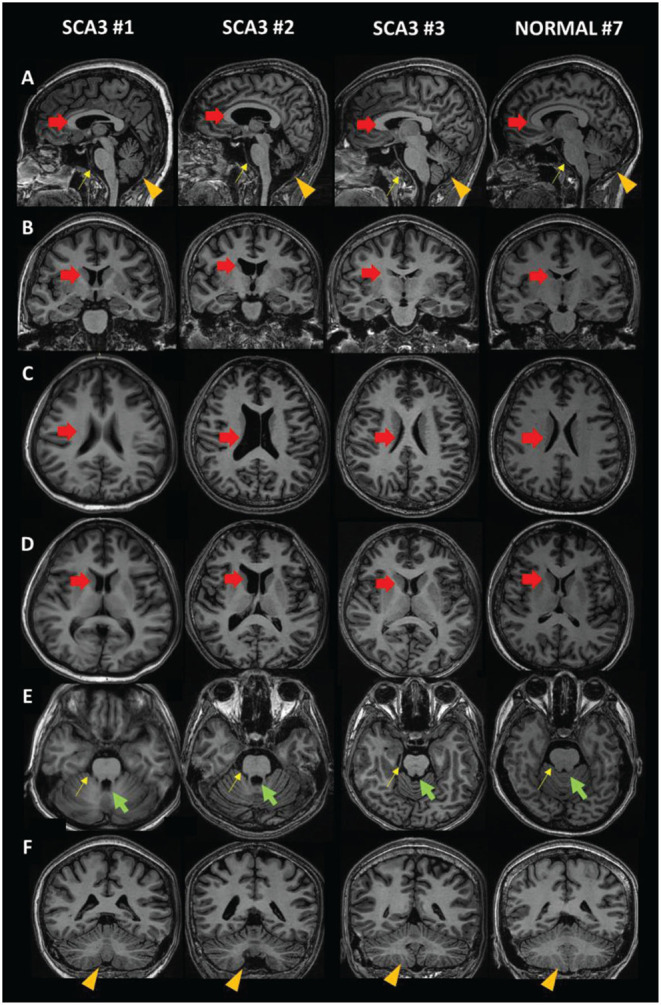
MRI images of SCA3 patients compared to a normal subject. (A) Sagittal view. (B,F) Coronal view. (C–E) Axial view. Red arrow: lateral ventricle (dilated in SCA3); green arrow: fourth ventricle (dilated in SCA3); yellow arrow: brainstem (size reduced in SCA3); orange arrowhead: cerebellum (size reduced in SCA3).
